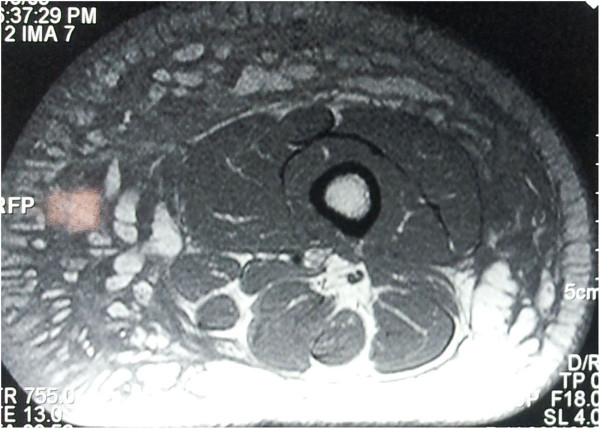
Magnetic resonance imaging scan of the thigh showing thick septate mass arising from subcutaneous tissue of anterolateral and medial aspects of the thigh without any contrast enhancement-transverse section.
